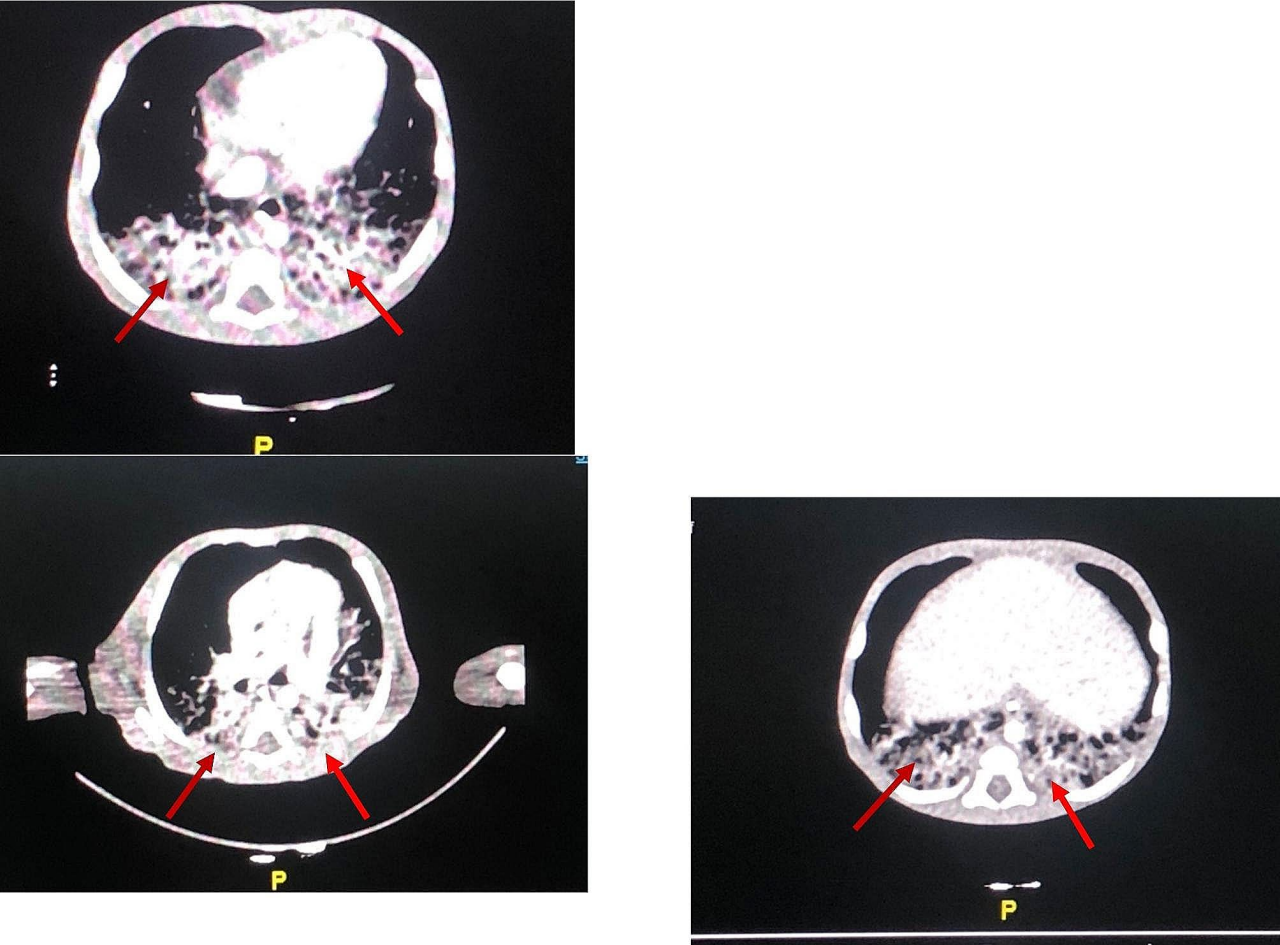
Chest computed tomography. Diffuse bilateral air space consolidation with air bronchogram seen more at the basal segment of both lower lung lobes
